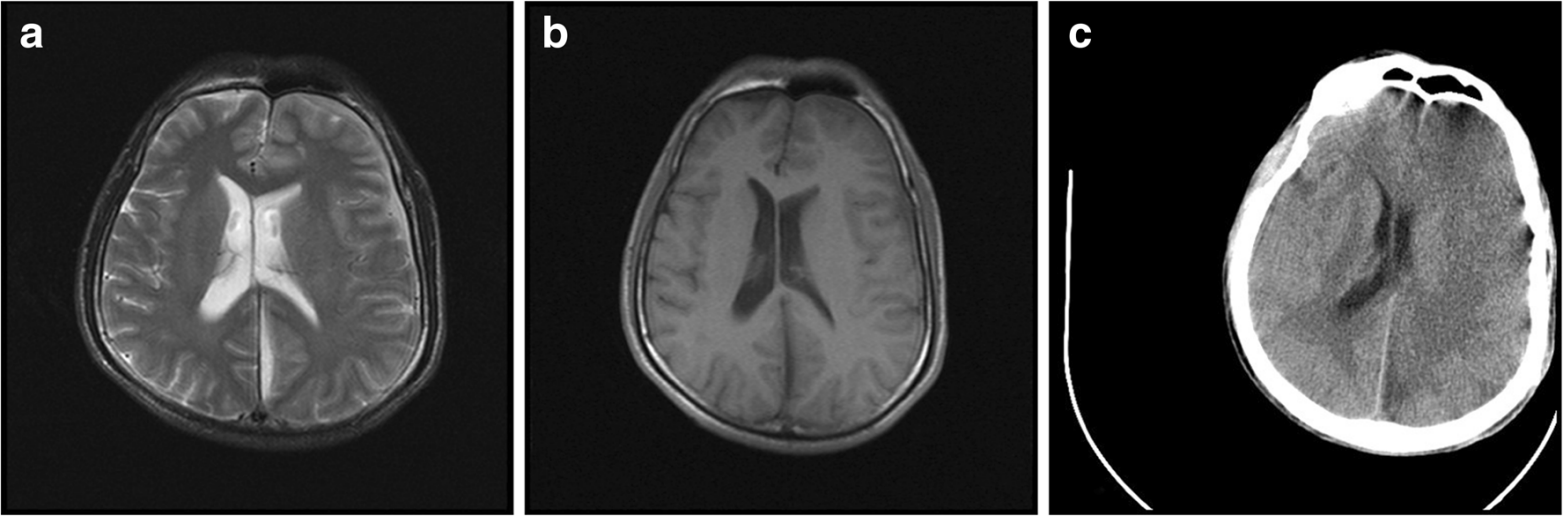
Compared with the pre-treatment MR(Fig. 2a T2WI, Fig. 2b T1WI), the post-treatment CT (Fig. 2c) revealed aggravated swelling of the left cerebral hemisphere, especially in cerebral cortex, shallowing of cerebral sulcus and schizencephaly, narrowing of the left lateral ventricle due to compression. The midline structure had shifted to the right obviously,which indicated cerebral hernia was developed
